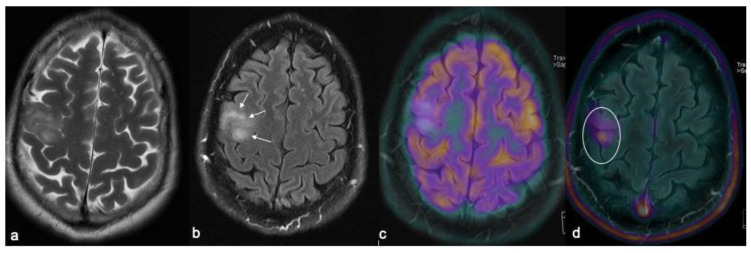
IDH-WT glioma with ill-defined borders in the posterior aspect of the middle frontal gyrus showing an inhomogeneous high signal on the T2w fast spin echo (a) and FLAIR (arrows in (b)) sequences with no radiotracer uptake at FDG-PET (c), but demonstrating a noticeable uptake upon FET-PET examination (circle in (d)).
